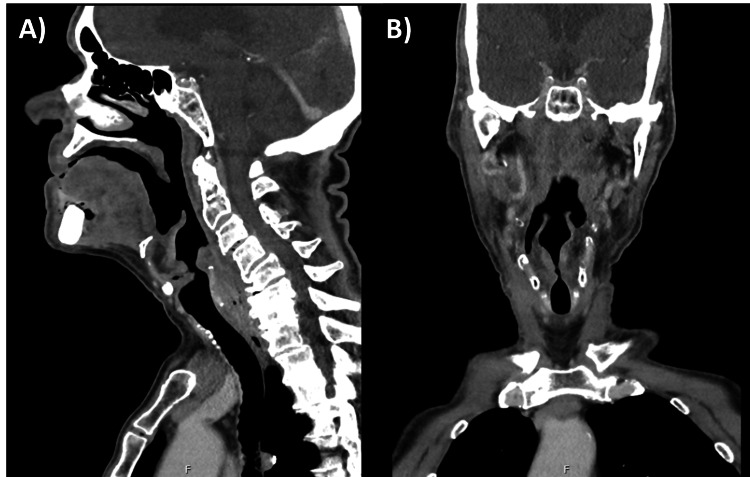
CT neck with contrast in sagittal (A) and coronal (B) planes demonstrating an upper oesophageal sphincter mass lesion with oedematous changes, suspicious for underlying malignancy.
